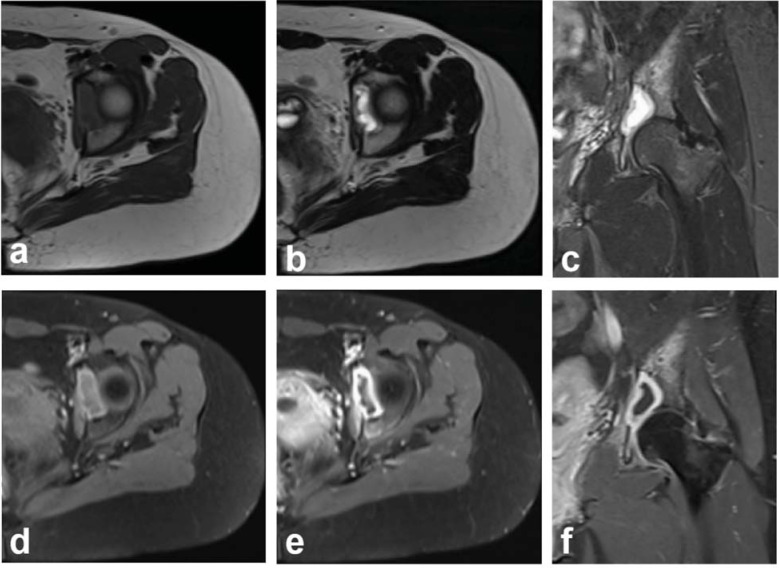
MRI of the left hip joint before treatment. (a) Axial image of T1-weighted image. (b) Axial image of T2-weighted image. (c) Coronal image of T2-weighted image. (d-f) Contrast-enhanced T1-weighted images. MRI showed irregular bone destruction with cortex erosion in the left acetabulum, the abscess showed a low signal intensity on the T1-weighted image and high signal intensity on the T2-weighted image,adjacent bone and soft tissue edema. The abscess wall was strengthened and the pus was not strengthened.
